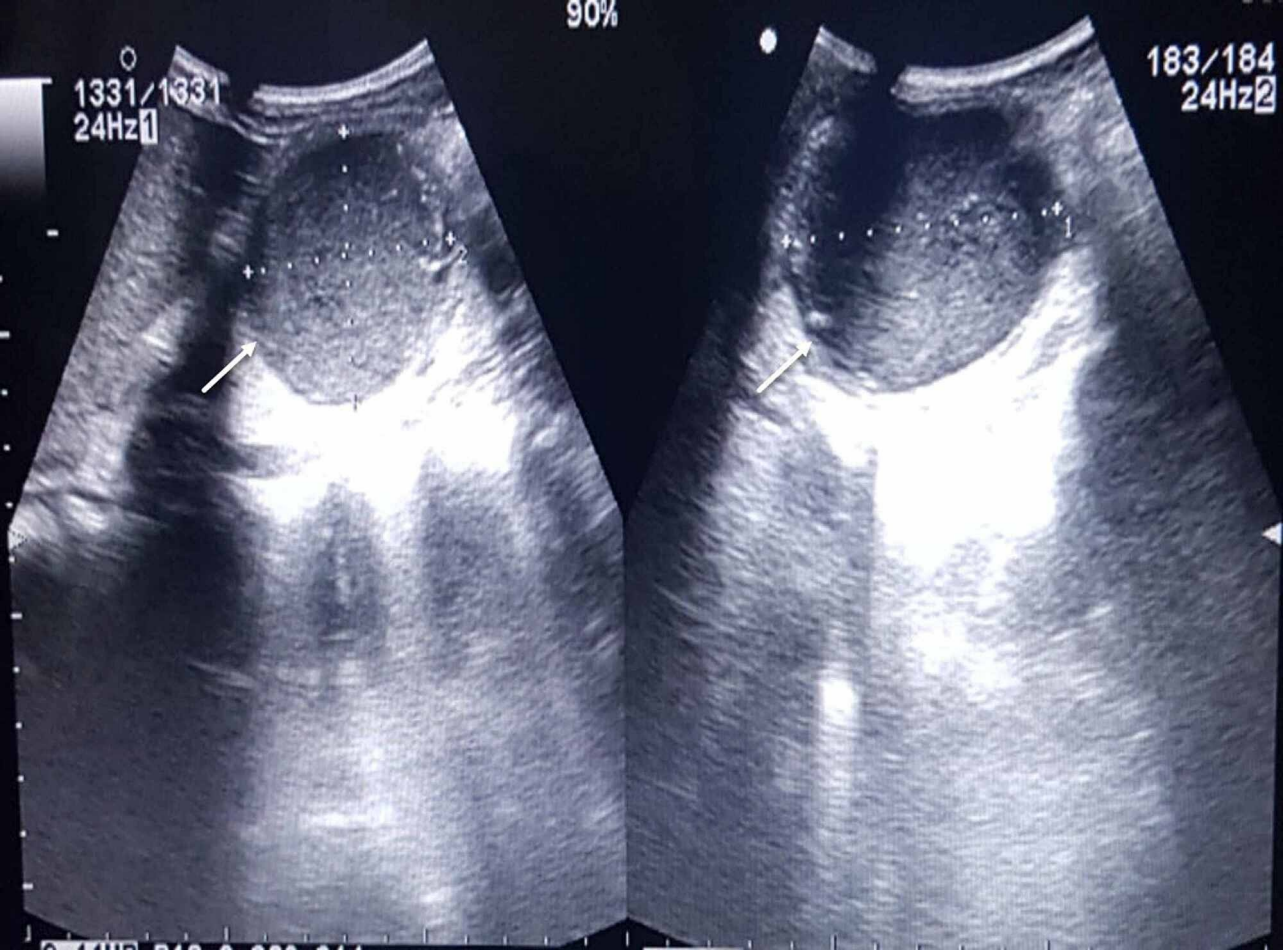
Ultrasonography by the convex probe, demonstrates the cystic lesion (White arrow), inferior to the liver.
